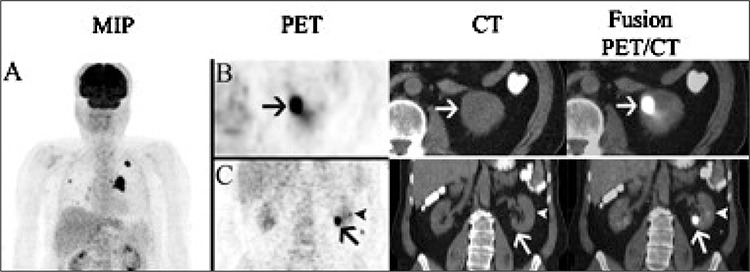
In baseline PET/CT, besides thoracic findings, multiple hypermetabolic bone and soft tissue lesions were observed (A). Although not reported, 2cortical lesions were seen in the lower pole (B-C, arrows) and middle zone (C-D, arrow heads) of the left kidney
